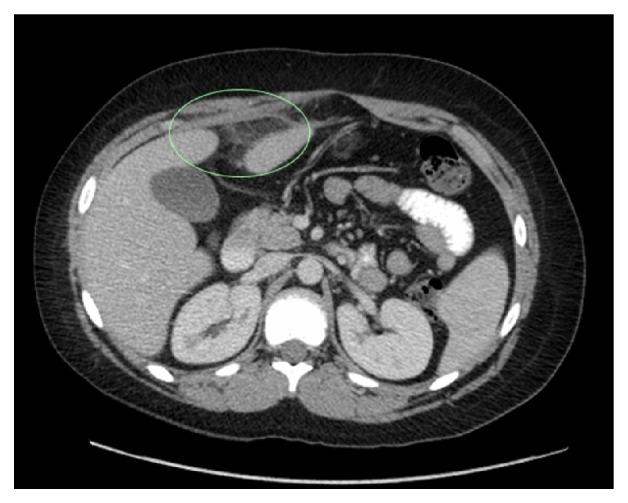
CT abdomen and pelvis, coronal view. Torsion of lipomatous appendage of falciform ligament circled.
